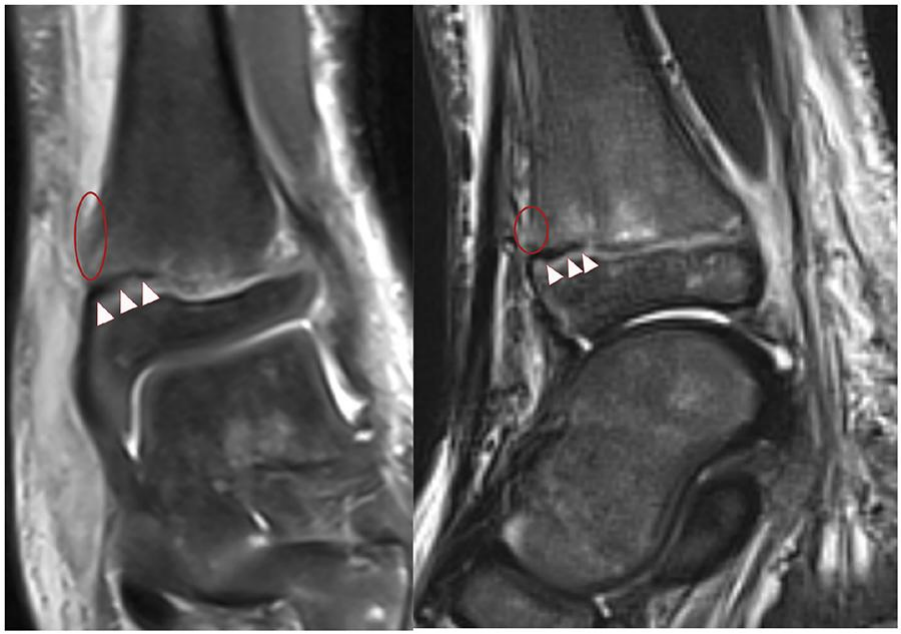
MRI showed periosteal entrapment in the anteromedial quadrant of the distal tibial physis (white arrow), along with a periosteal defect in the metaphysis (red circle).
